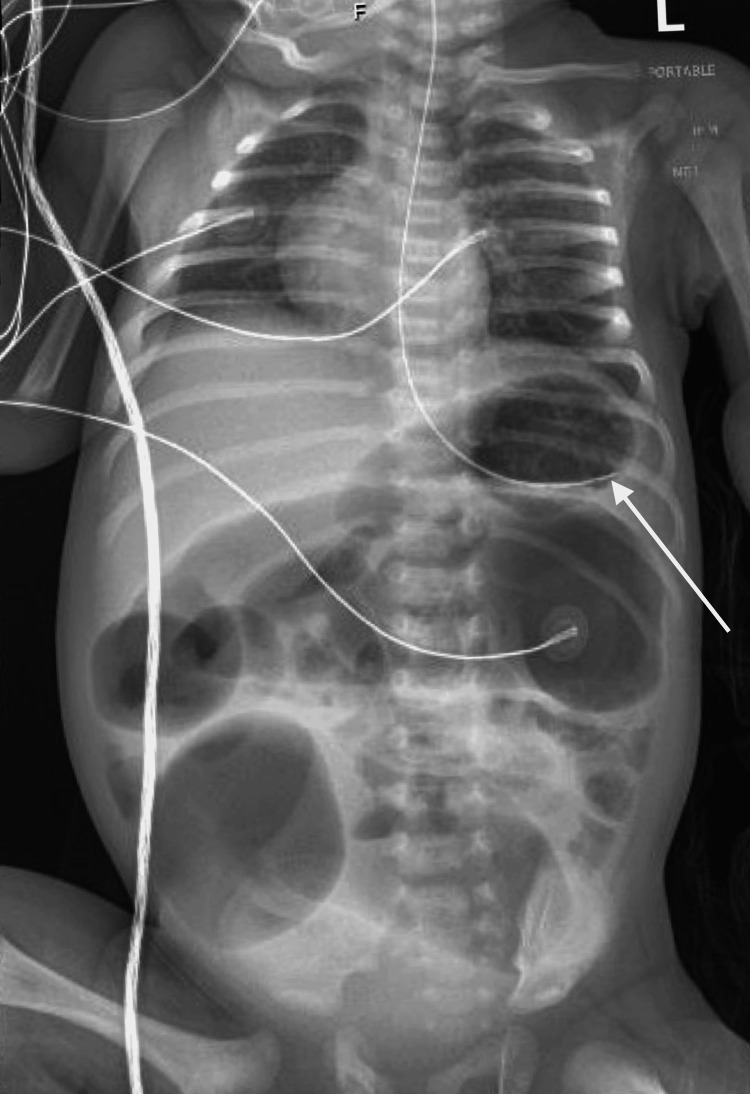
One-view X-ray abdomen taken on day 3 of the second admission The arrow points to the tip of the enteric tube inside the stomach. Overall impression: Interval placement of an enteric tube that follows the expected course of the esophagus. The tip overlies the stomach bubble.
